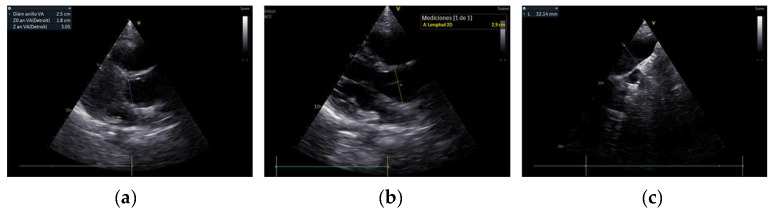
Ascending aortic dilatation in individual I2. (a) Parasternal long-axis view of the aortic ring, 25 mm (z + 3.27). (b) Sinus of Valsalva, 29 mm (z + 1.5). (c) Suprasternal view of aortic arch with ascending aorta, 30 mm (z + 2.94).
